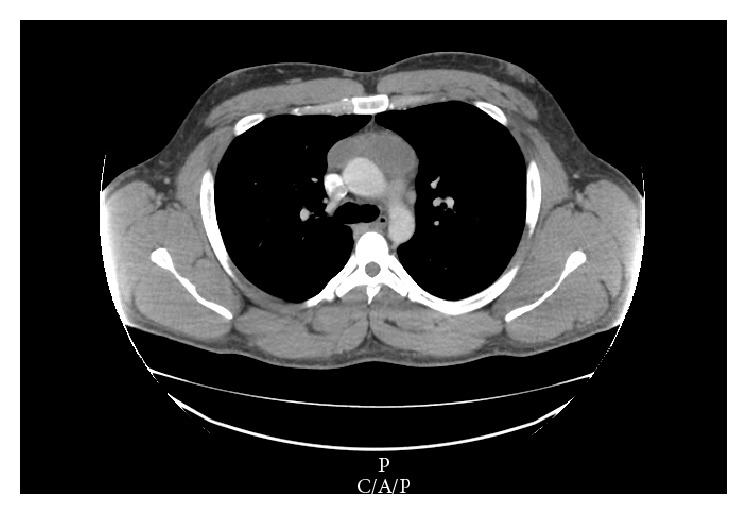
Computed tomography scan showing a 7.6 × 2.6 cm anterior mediastinal mass.
